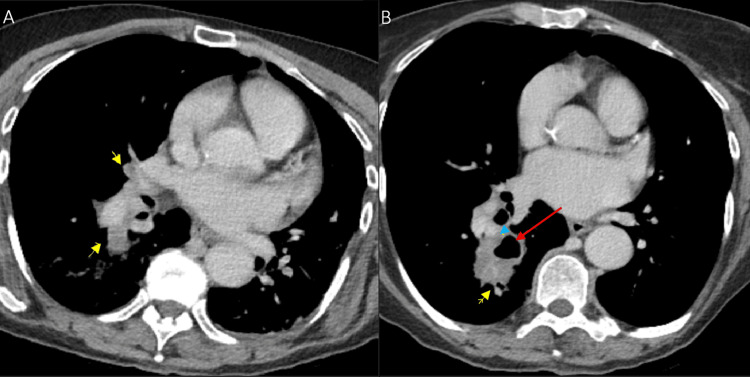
Axial contrast-enhanced computed tomography (CT), soft tissue window. (A) Multiple filling defects predominantly in the right pulmonary arteries representing pulmonary embolism. (B) Three weeks later: hypodense consolidation of the right lower lobe (yellow short arrow) with internal cavitation (red long arrow), centered upon an occluded pulmonary artery branch (blue arrowhead), concerning for septic pulmonary embolism or cavitary pulmonary infarction with superimposed infection. Interval improvement of most of the pulmonary embolism burden elsewhere, with residual linear streaks suggesting chronicity.
